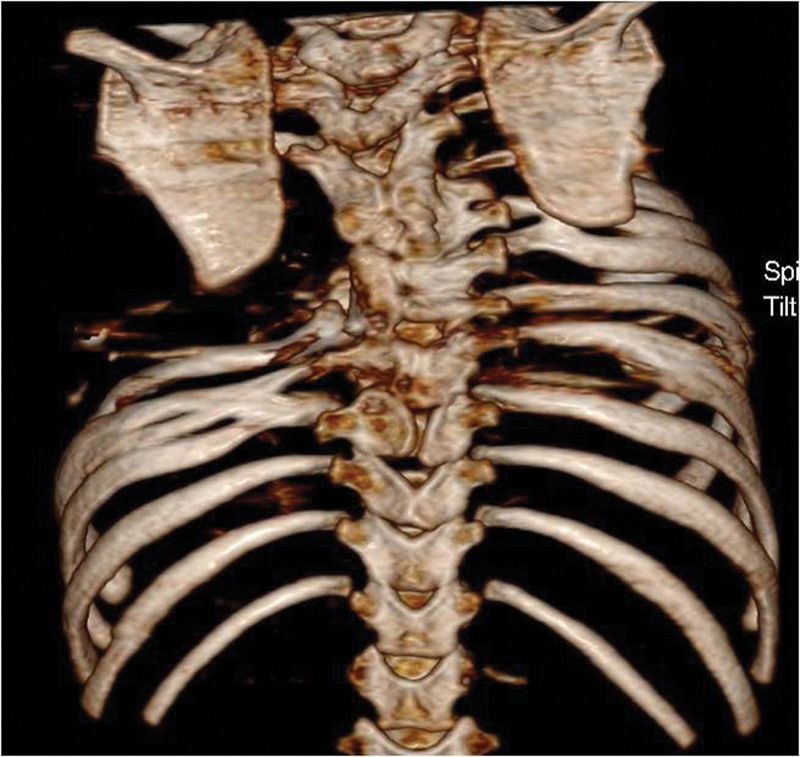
3D CT reconstruction image of the chest and thoracic spine (posterior
